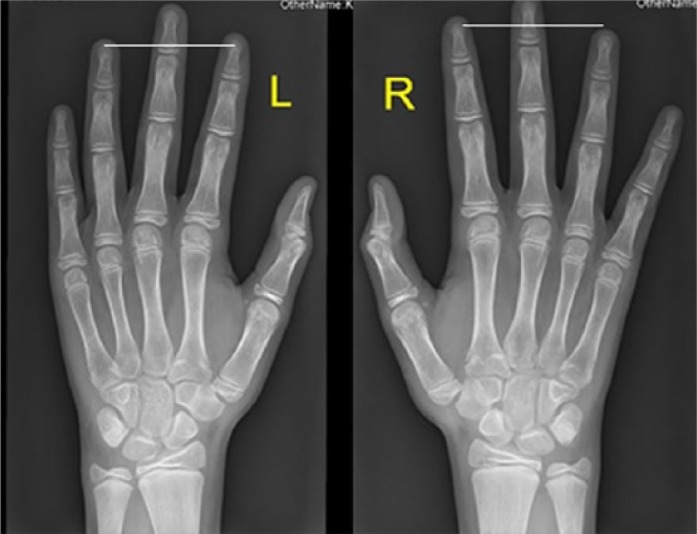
AP hand radiographs in a 13-year-old boy with Klinefelter syndrome showed phalangeal preponderance (normally the length of the fourth metacarpal is equal to length of distal plus proximal phalanges).
